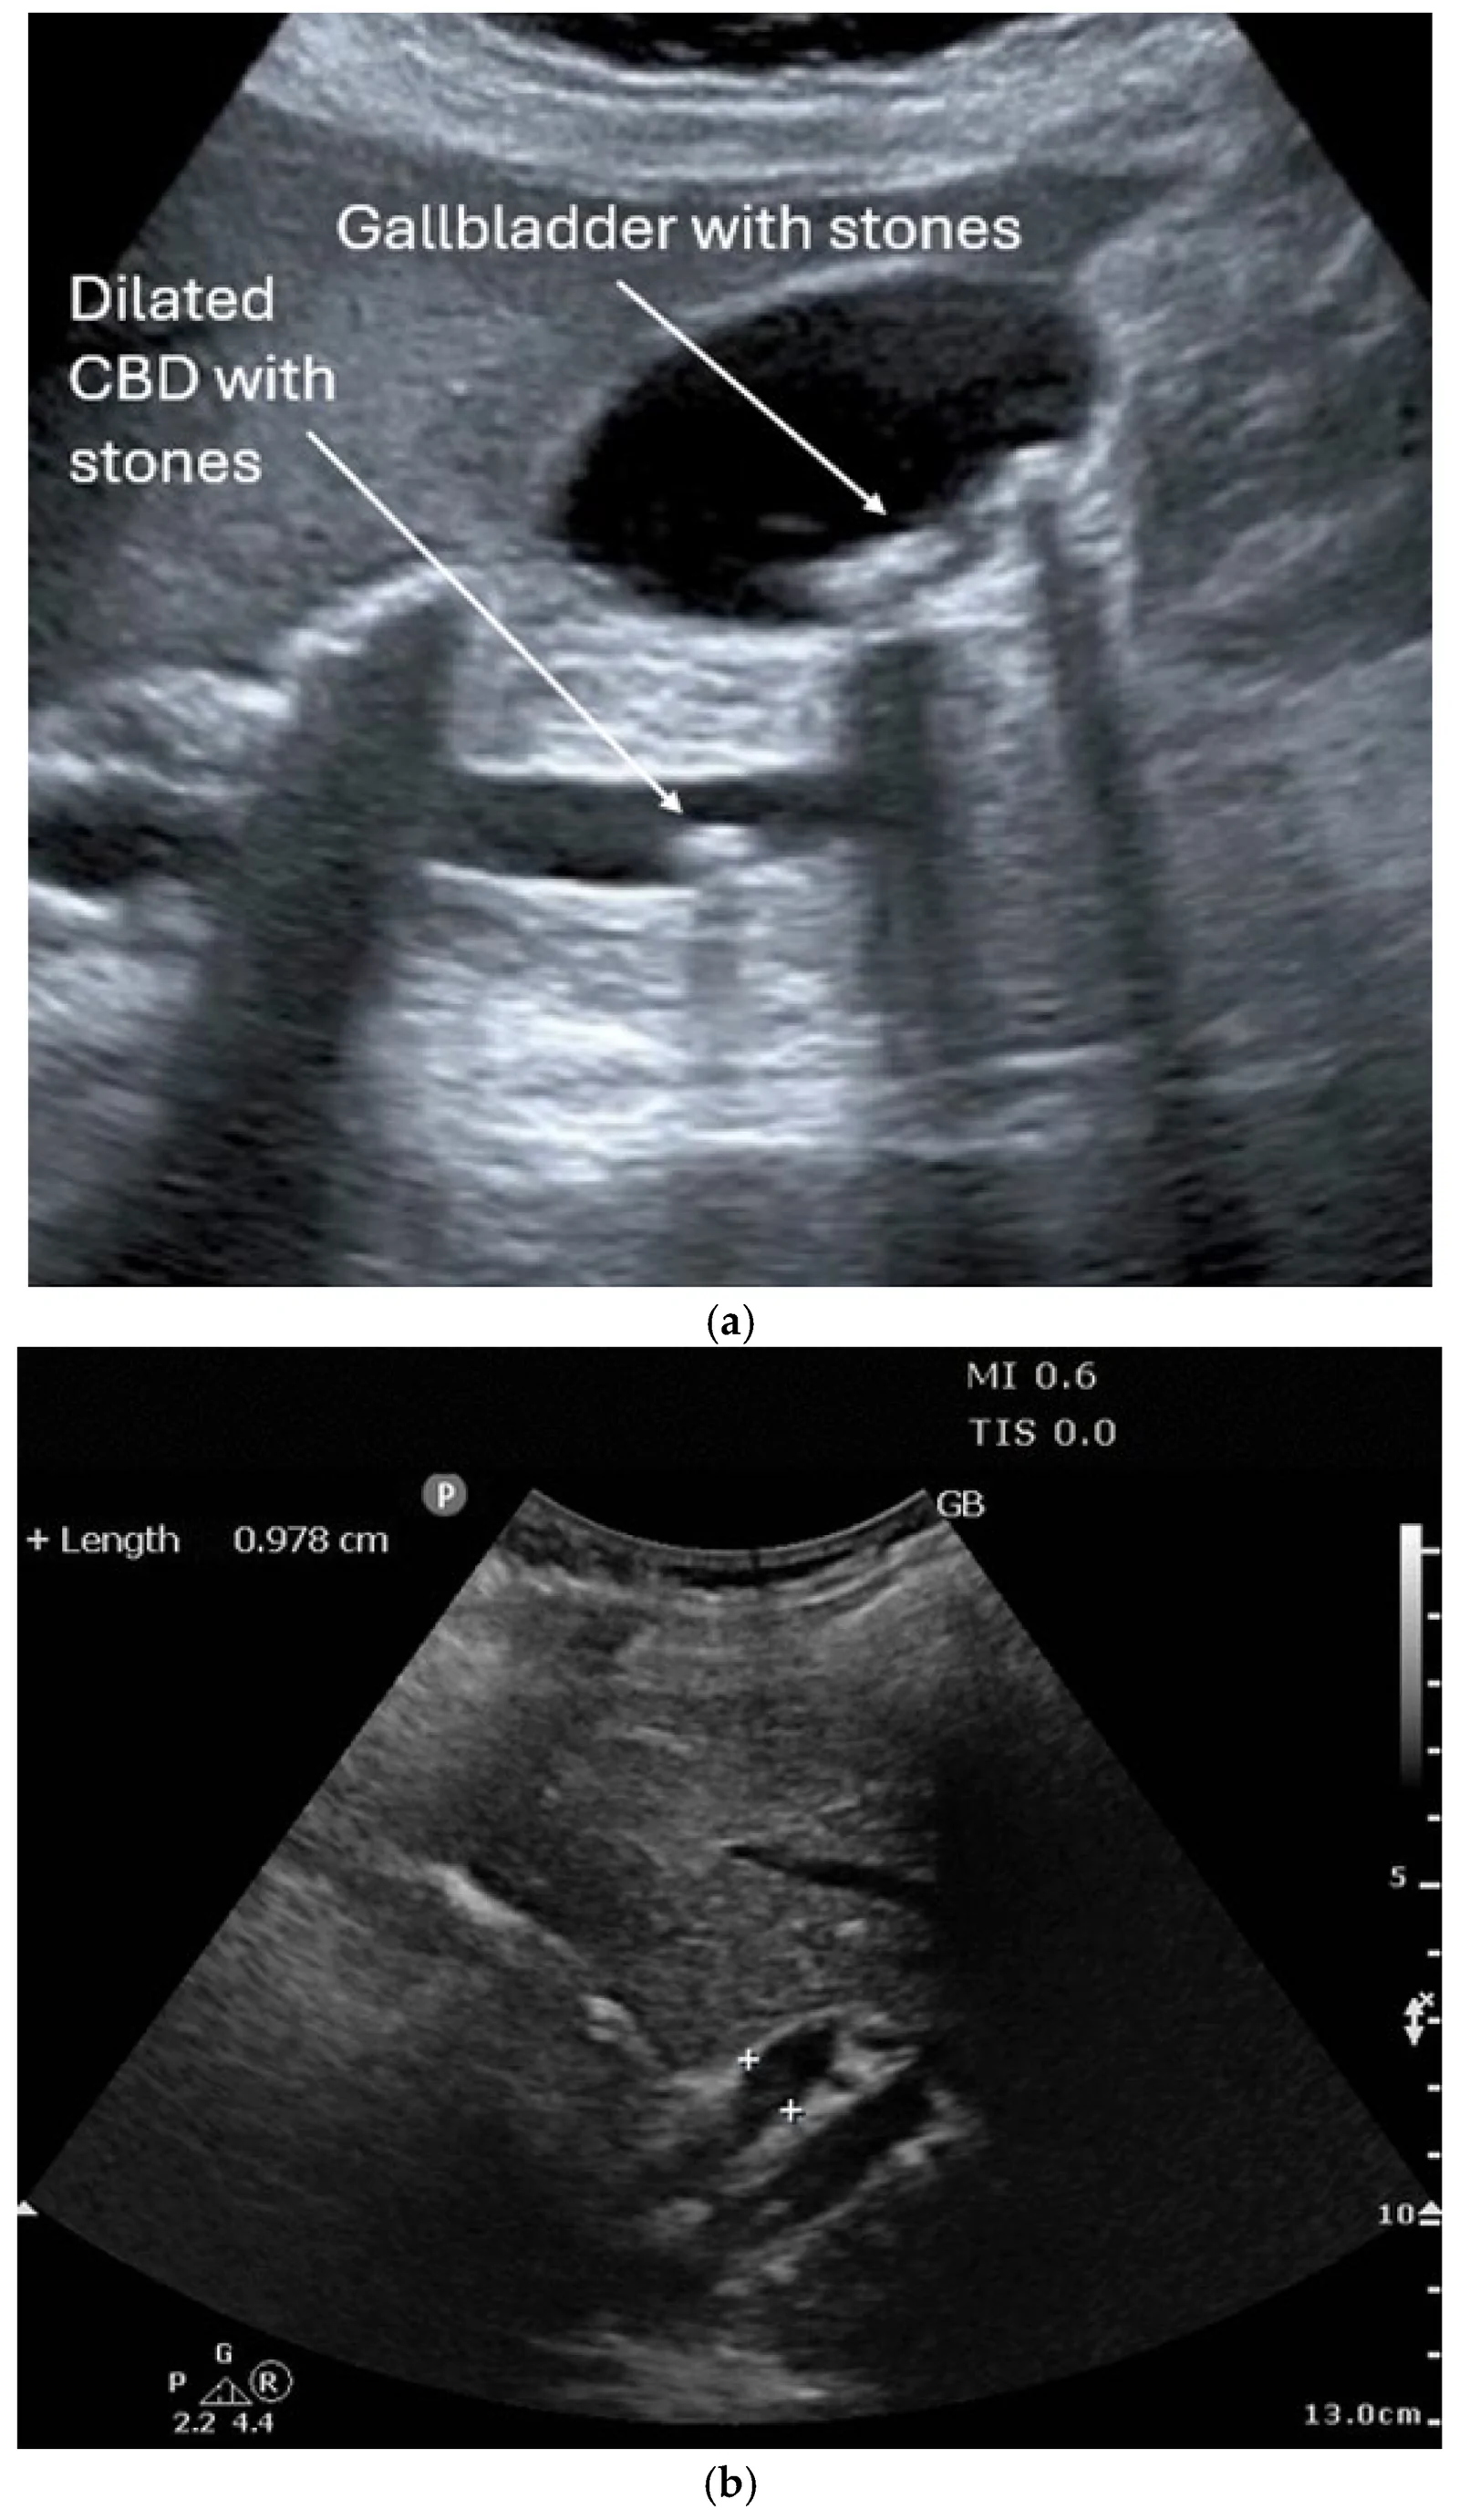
(a,b) Gallstones can escape the gallbladder through the ductal system and cause choledocholithiasis, which refers to stones within the CBD. Patients often present with colicky RUQ abdominal pain, with possible jaundice, pruritis, nausea, and vomiting. When assessing for choledocholithiasis, POCUS should be performed both longitudinally and transversely through the common bile duct. Radiology defines a dilated CBD as >6 mm. For POCUS users, a practical reference for calculating a normal CBD diameter is 3 mm plus 1 mm for every decade in age over 30 years old, although this is not an absolute diagnostic threshold [3,4]. Exceptions to this rule include post-cholecystectomy patients, whose CBD may measure up to 10 mm and patients with older age, who may have a higher CBD measurement. Potential CBD findings include visualization of intraluminal stones as hyperechoic round structures ranging from 2 mm to over 20 mm [3,4,16,17]. Users should note that gas in the duodenum may complicate stone detection, so they should be cautious with a negative POCUS exam but high clinical suspicion. The common bile duct and intrahepatic biliary tree may be dilated without a visualized stone. Additionally, multiple stones in the gallbladder may be visualized, which should increase suspicion for choledocholithiasis. In these cases, particularly if the patient has transaminitis or an elevated lipase level, additional workup should be considered such as a RUQ radiology ultrasound. A CT scan can be considered for a comprehensive view of the gallbladder and for ductal dilation, plus complications such as pancreatitis or adjacent abscess. Gastroenterology should be consulted for potential intervention with Endoscopic Retrograde Cholangiopancreatography (ERCP) to remove the stones or for Magnetic Resonance Cholangiopancreatography (MRCP) to visualize and confirm their location. Complications from choledocholithiasis can include ascending cholangitis, appearing as bile duct wall thickening on POCUS. In cases of ascending cholangitis, the patient can become critically ill quickly. Thus, they should be treated emergently with intravenous antibiotics to prevent serious complications and mortality from infection dissemination [3,4,16,17]. Furthermore, patients with prior cholecystectomy or RUQ abdominal surgery can develop post-operative biliary obstruction. This can sometimes be visualized on ultrasound as biliary ductal dilation with possible extension into the hepatic ducts. Post-operative cholecystectomy patients can have normal CBD dilation up to 10 mm. Recent surgery warrants general surgery consultation and CT imaging for further clarity, as POCUS performance in the post-operative patient is very limited [3,4,16,17]. Due to post-surgical changes in anatomy, cross-sectional imaging should be prioritized in these patients. CT can provide a rapid assessment of post-operative anatomy and complications. Whereas, magnetic resonance imaging (MRI)/MRCP offers a more detailed view of the biliary and pancreatic ductal systems [17,18].
